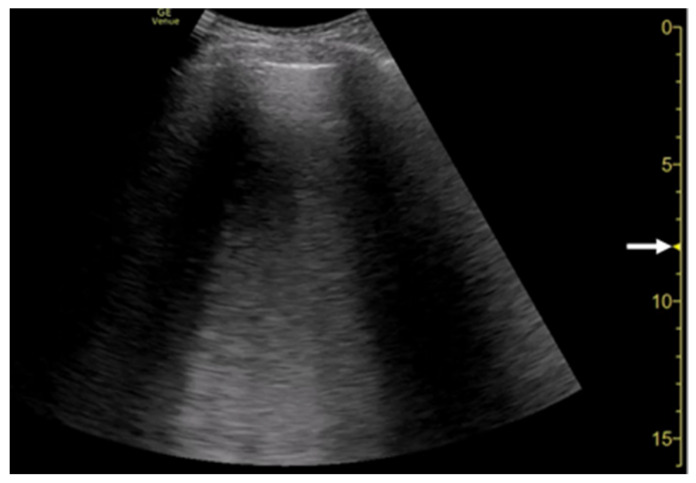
Ultrasound image obtained by Venue scanner (GE Healthcare) with a curvilinear transducer in the same case as Figure 5. As the single focal point (arrow) is shifted to a deeper level, each B-line becomes wider and overlaps each other.
